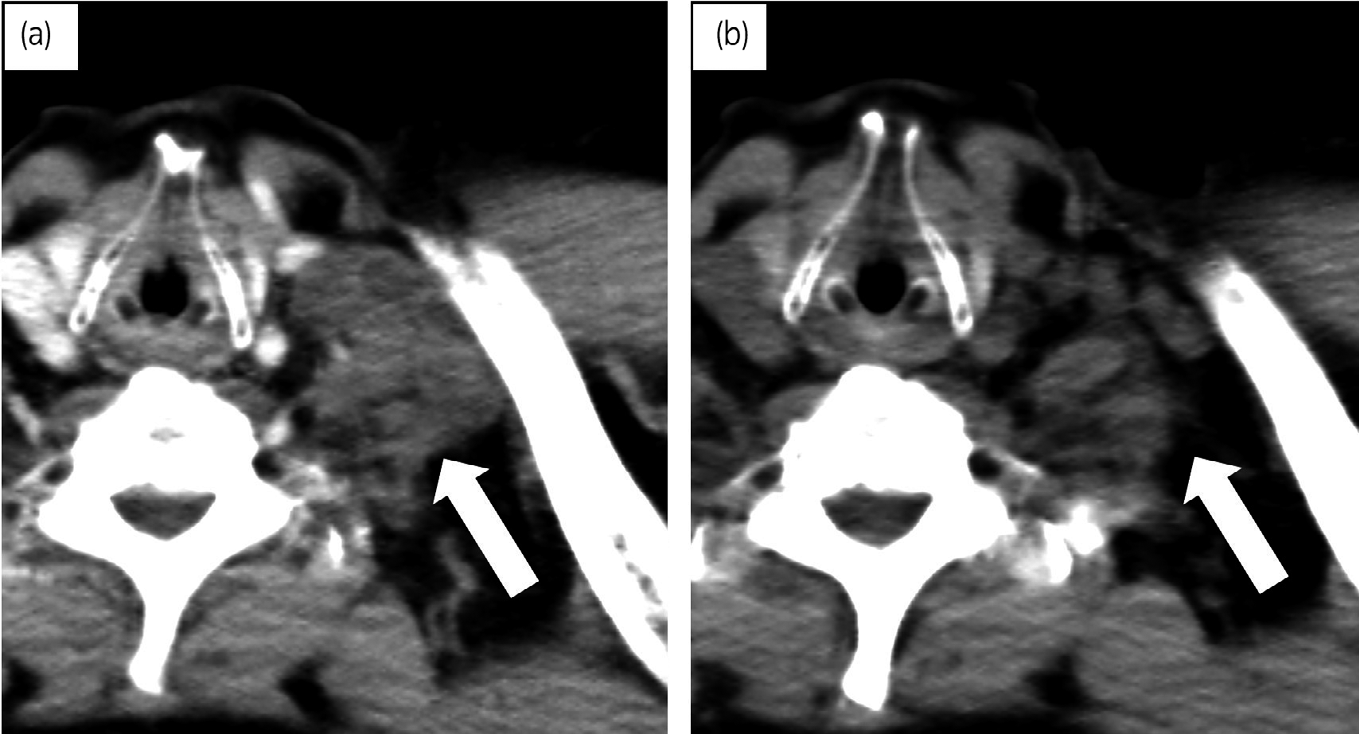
CT images before initiation of docetaxel (a) and after two courses of docetaxel (b) showing remarkable shrinkage of swollen left supraclavicular lymph nodes (arrow).
